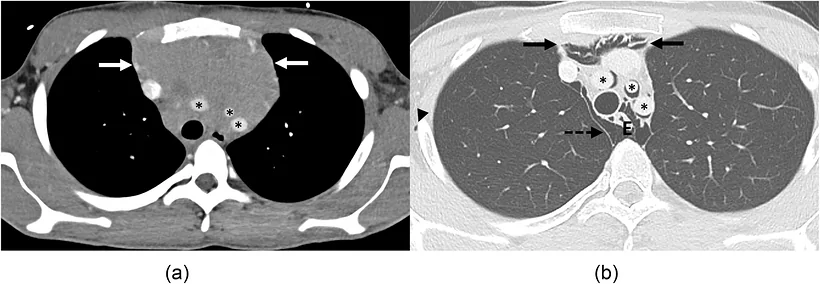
(A) Axial CT image (soft tissue window) obtained at time of diagnosis shows a large solid mass centred in the anterior mediastinum (white arrows) with encasement of the major arch vessels (*). (B) Follow‐up axial CT (lung window) obtained 1 month later depicts resolution of the mediastinal mass with new widespread pneumomediastinum, within the anterior mediastinum (black arrows), surrounding the arch vessels (*), encasing the oesophagus (E), throughout the posterior mediastinum (dashed arrow) and dissecting into the right lateral chest wall (arrowhead).
